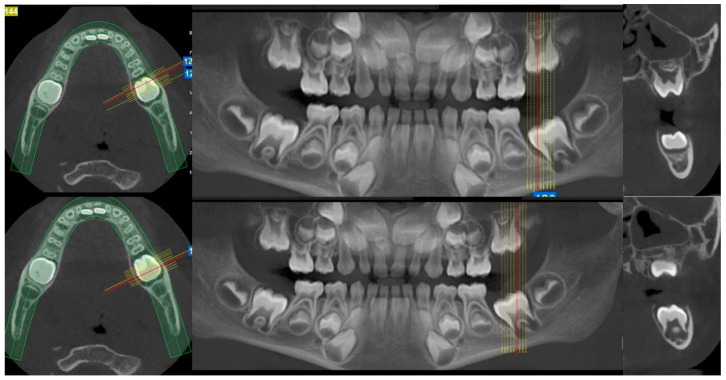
The CBCT examination did not reveal any bone involvement.
